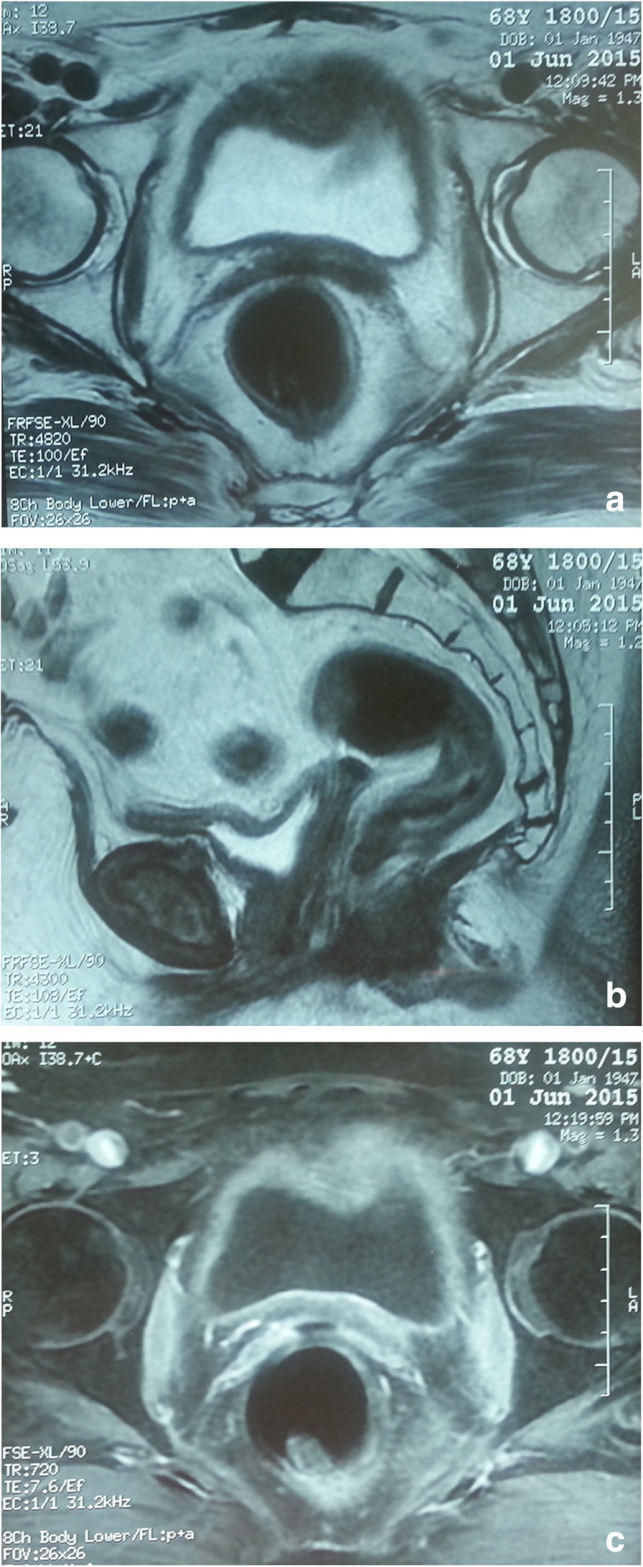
a–c Axial (a, c) and sagittal (b) MRI images showed a complete response 6 months after completing the treatment
